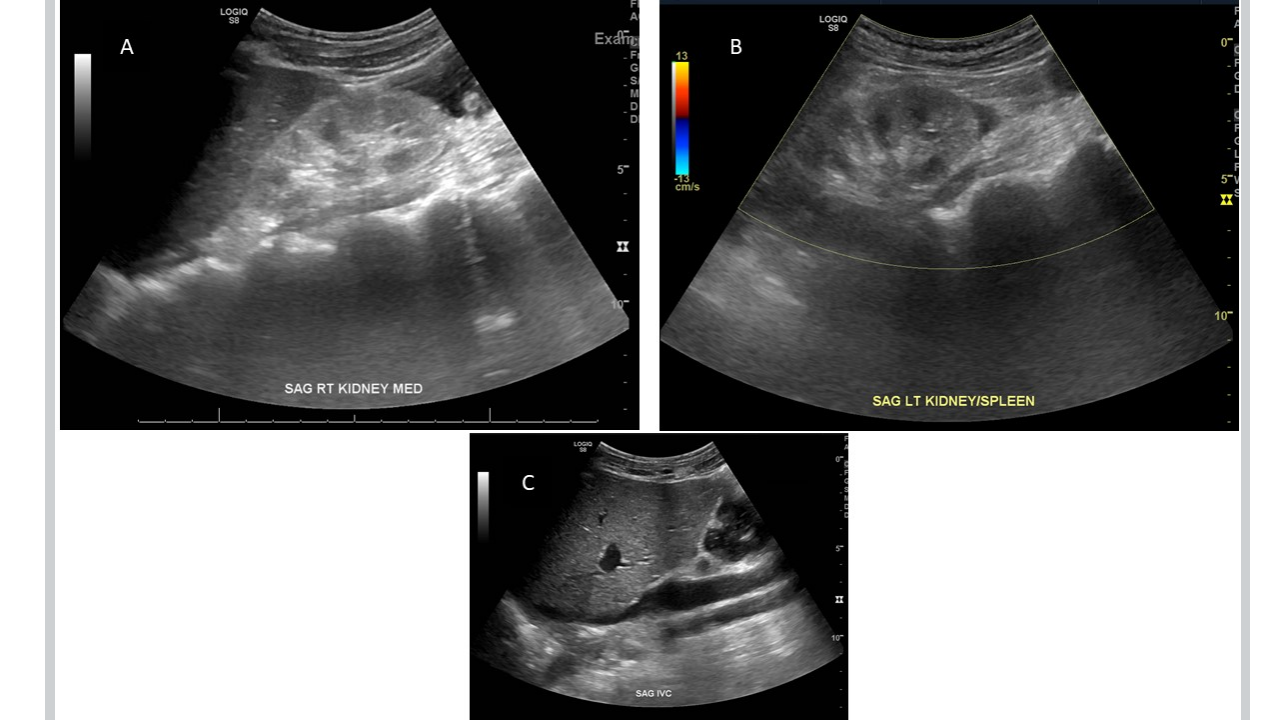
Bilateral Kidney and IVC: Case 1. A) The right kidney appears more echogenic compared with the adjacent liver and has increased medullary pyramid differentiation consistent with ATN. B) Left kidney also with increased echogenicity and differentiation. C) Liver, IVC, and aorta in view. IVC collapsibility index >50%. JVP on ultrasound also found to be at the level of the clavicle (not shown).
